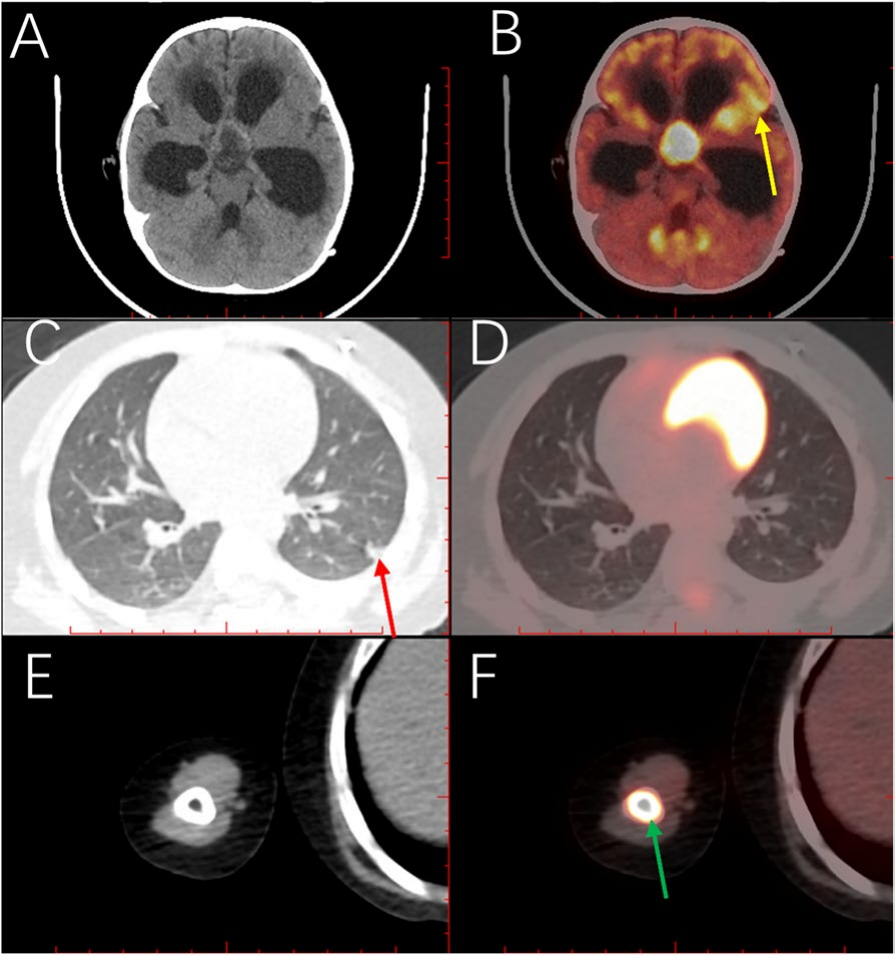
The whole-body positron emission tomography-computed tomography (PET-CT). PET-CT scan shows disseminated lesions involving multiple organs, including the brain (B, yellow arrow), the lung (C, red arrow), and the right humerus (F, green arrow). The measured resolution for images was 360 ppi
